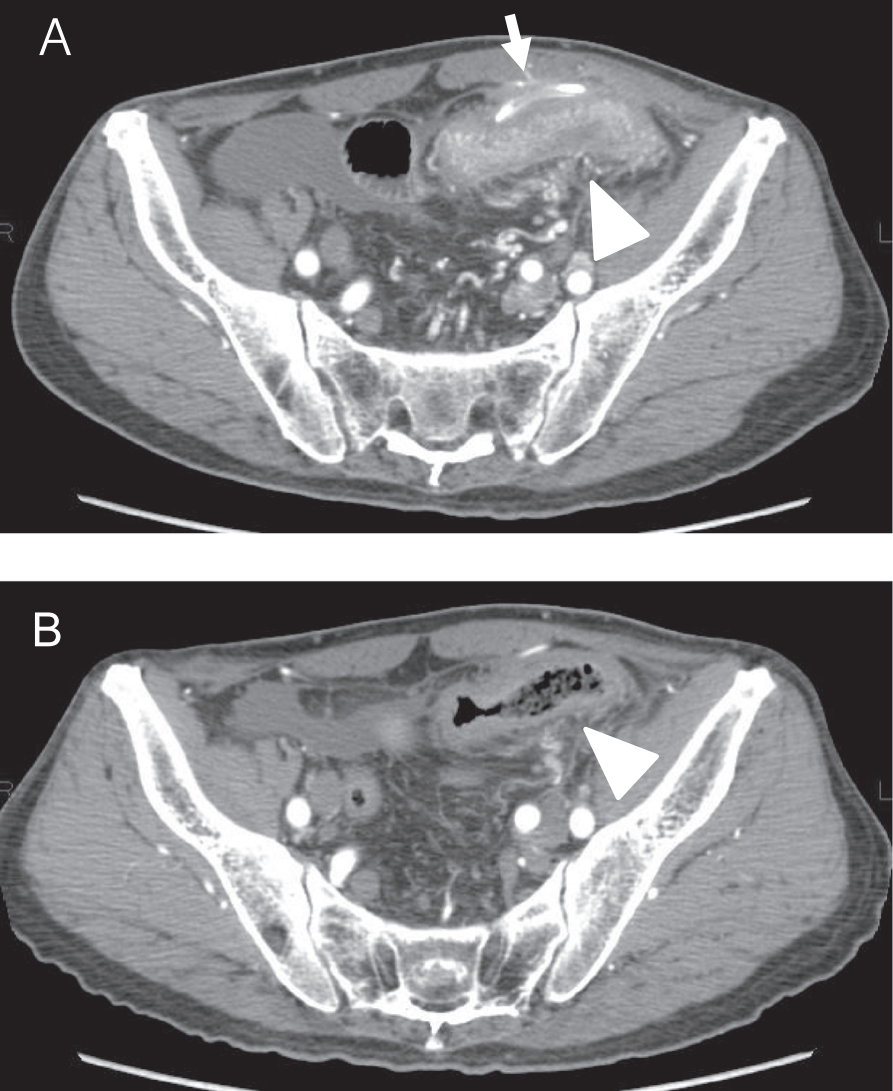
Computed tomographic changes in sigmoid colon before and after removal of intraperitoneal access port system. (A) A computed tomography scan showed marked wall thickening of the sigmoid colon (closed triangle) adjacent to the catheter (arrowhead) before removal. (B) Seven days after port removal, the wall thickening of the sigmoid colon had improved (open triangle).
